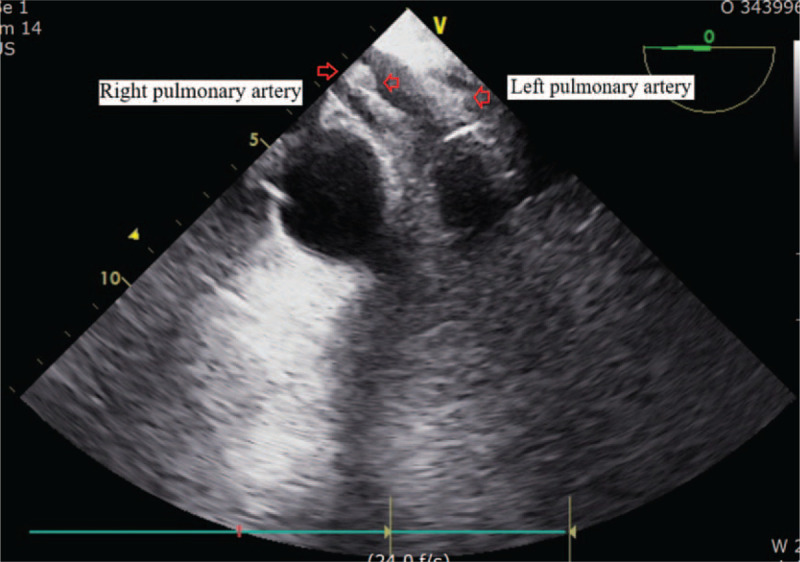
Multiple, huge thromboembolic clots in the right and left pulmonary artery shown by transesophageal echocardiography. Empty arrows indicate thromboembolic clots.
